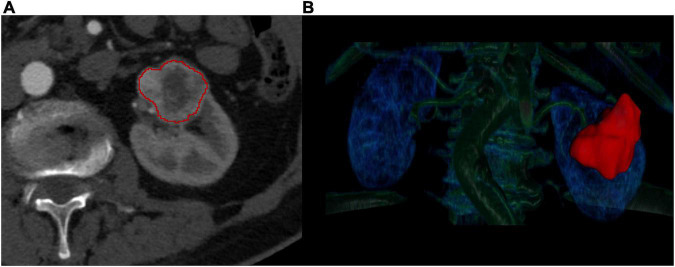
Manual segmentation of kidney tumors. The manual segmentation of the kidney tumors was completed on the corticomedullary phase axial CT scans (A). The entire lesion volume was delineated slice-by-slice (B) in order to perform a three-dimensional radiomics analysis.
